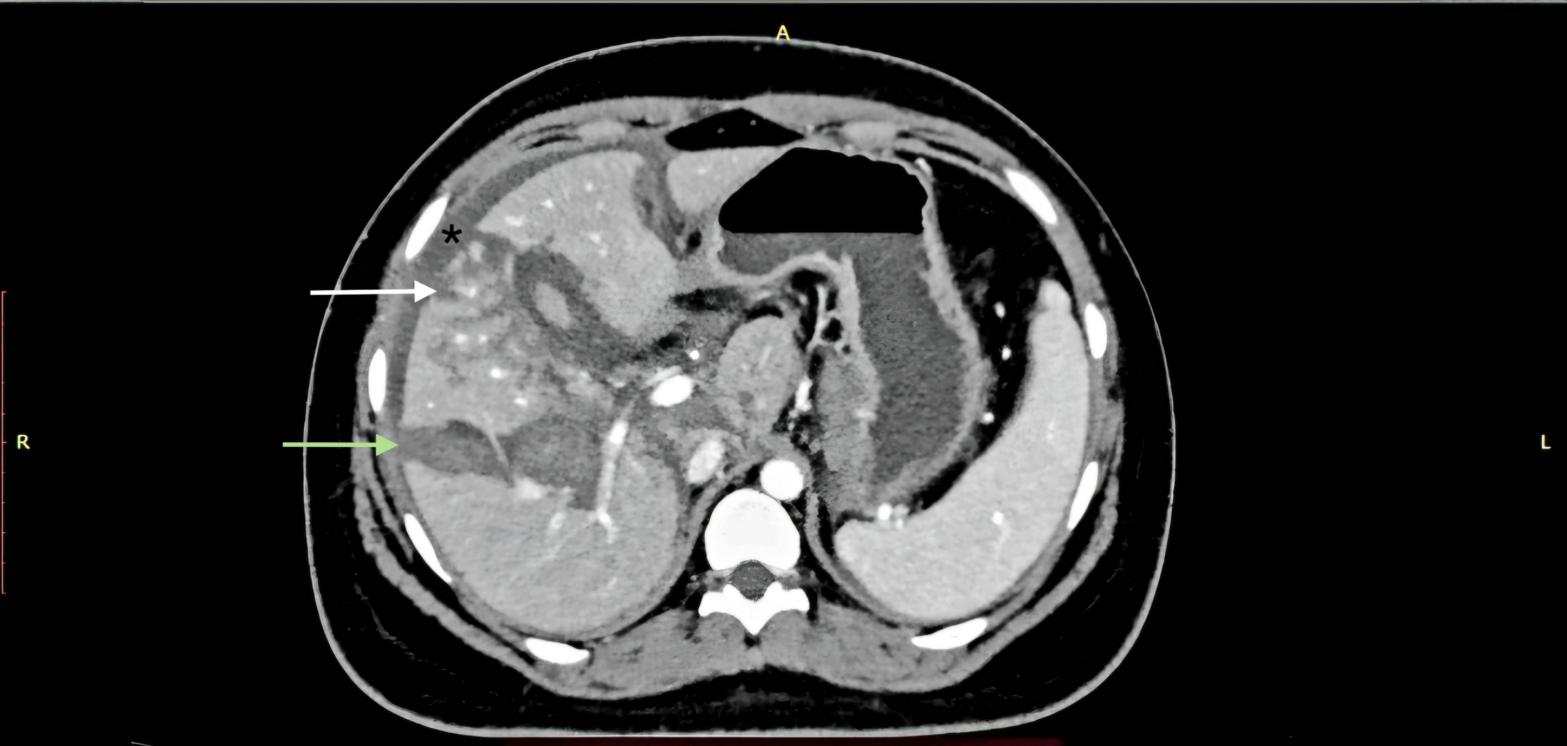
AAST grade IV liver injury showing parenchymal laceration with subcapsular hematoma on CECT (SB protocol). A 19-year-old female with a history of RTA underwent CECT of the abdomen using the SB protocol. The axial CECT image shows a large hepatic parenchymal laceration involving segments V (white arrow) and VIII (green arrow), as well as a subcapsular hematoma (black star), without active contrast extravasation. Findings were consistent with the AAST grade IV liver injury. AAST: American Association for the Surgery of Trauma; CECT: contrast-enhanced computed tomography; SB: split-bolus; RTA: road traffic accident.
